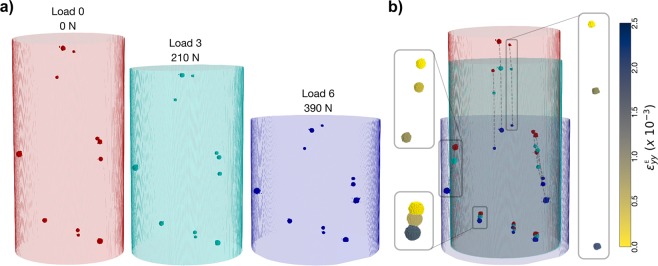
(a) Using custom python scripts, cubic iron oxide spheres were isolated from the full *μ*CT reconstruction and are shown for Loads 0, 3 and 6. (b) The movement of the grains are tracked across the three load steps and the insets show the corresponding elastic strains along the loading direction. An opensource 3D visualization software, ParaView 5.5^73^ was used to view the reconstructions.
